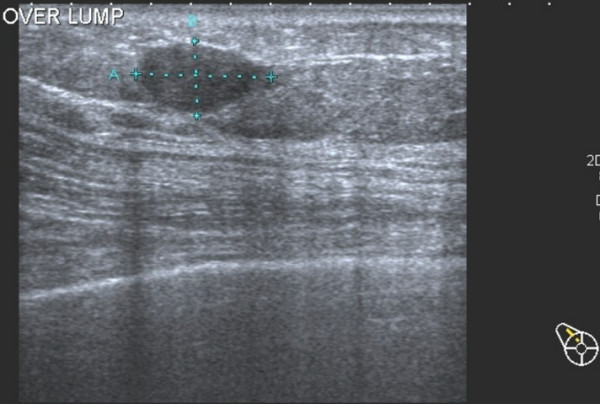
Ultrasonography showing hypoechoic mass in the right breast.
